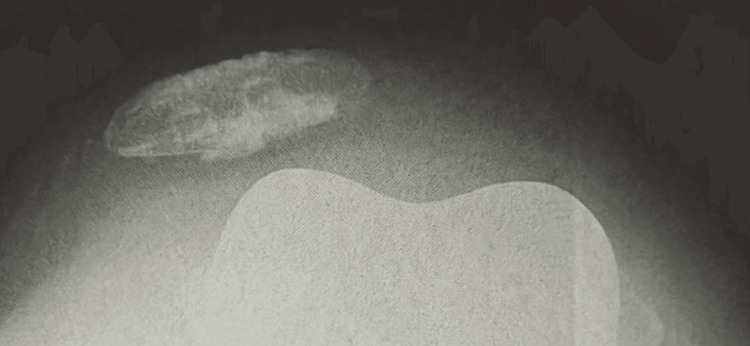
Postoperative skyline radiograph of a 67-year-old male suffering from repetitive patellar dislocations due to the internally rotated femoral component
